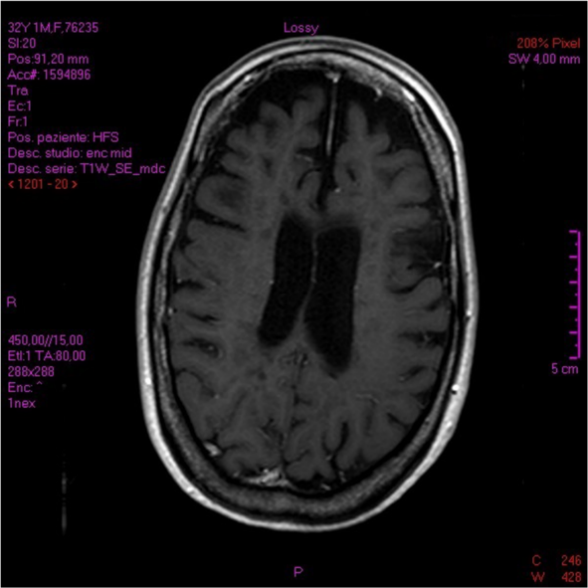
The post- treatment MRI check-up, performed 1 month after SIA, evidenced total regression of CELs
